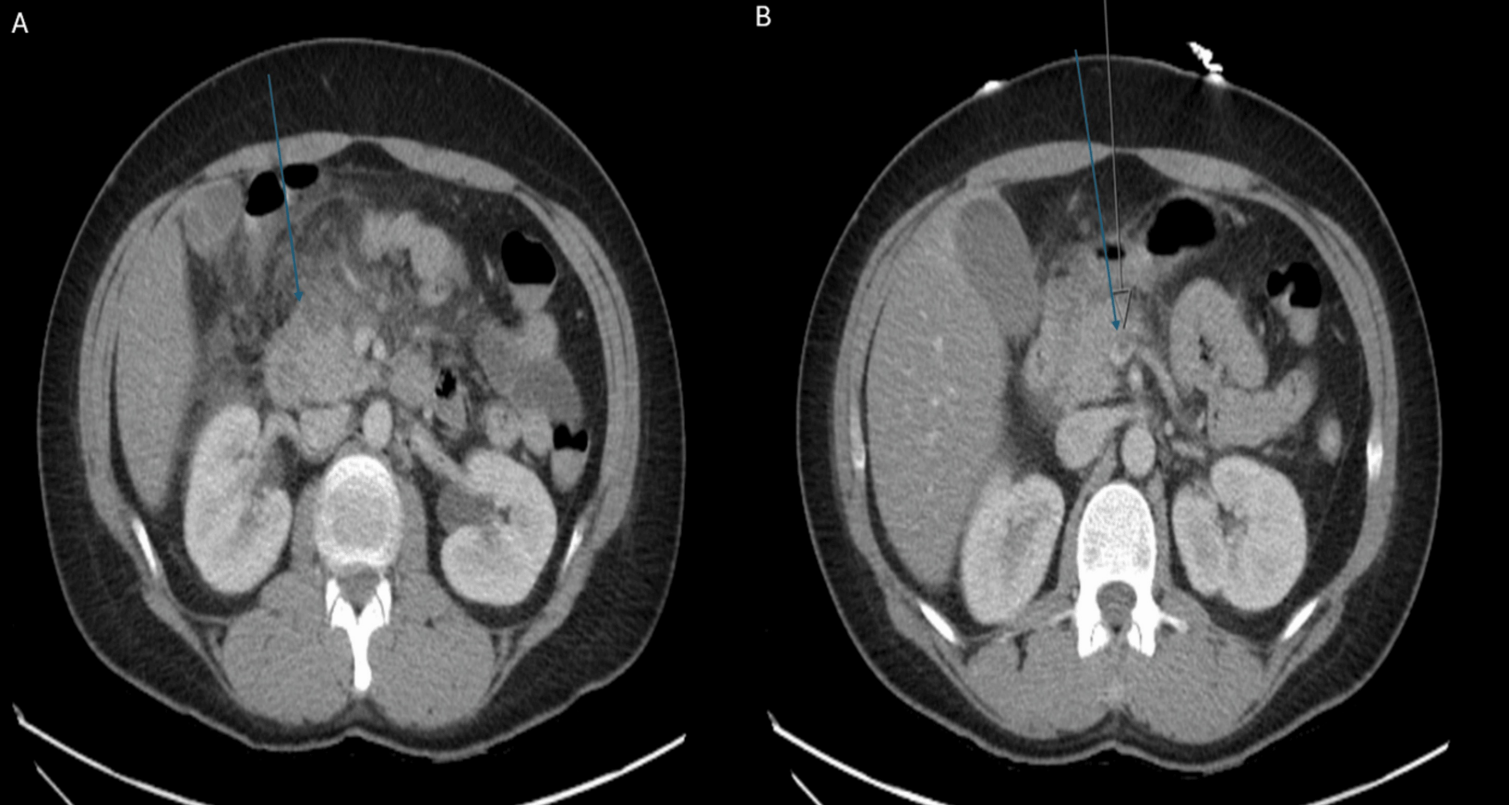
Contrast-Enhanced CT of the Abdomen Demonstrating Acute Pancreatitis and Associated Vascular Complication Contrast-enhanced CT of the abdomen demonstrating inflammatory and vascular complications of acute pancreatitis. (A) CT image showing marked inflammatory enlargement of the pancreatic head with surrounding peripancreatic fat stranding and edema, consistent with acute interstitial pancreatitis. (B) CT image from the same study demonstrating a hypodense filling defect within the superior mesenteric vein, consistent with acute thrombosis.
